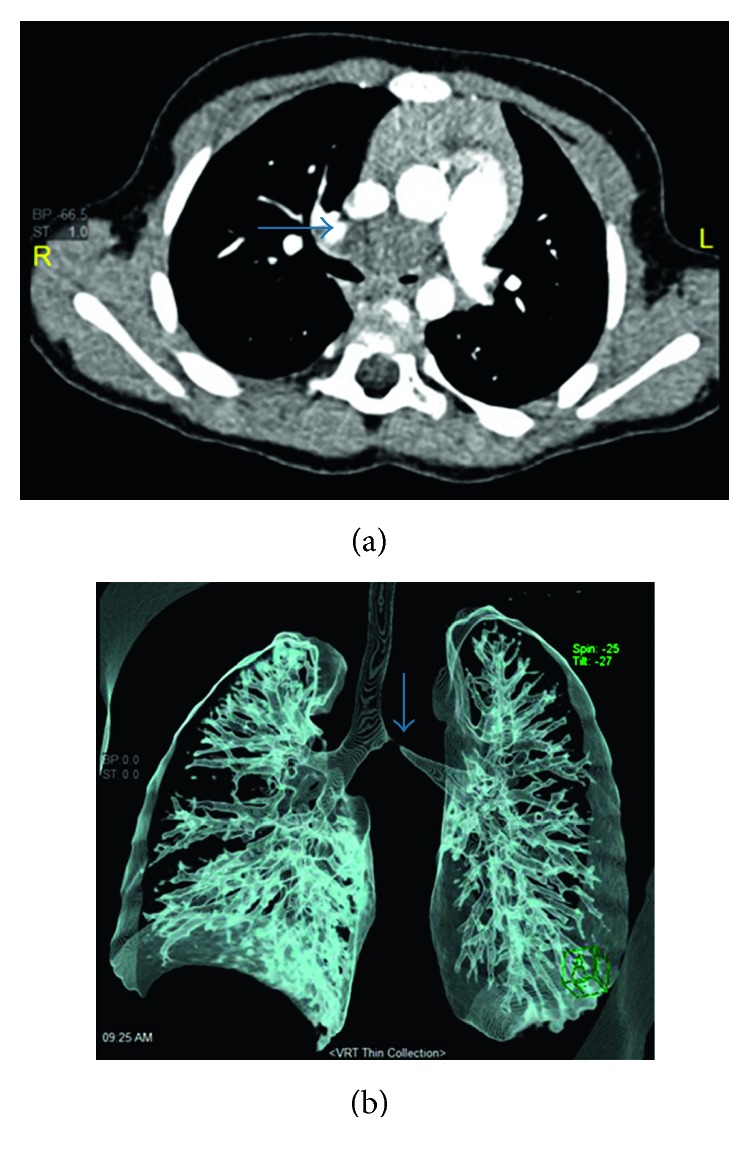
(a) CT scan of the chest showing a hypodense cystic lesion in the middle mediastinum (arrow), causing compression on the carina and the proximal aspect of the mainstem bronchi, more pronounced on the left main bronchus. (b) 3D reconstruction of the CT scan showing a significant narrowing of the left main bronchus (arrow), caused by external compression of the adjacent cystic lesion.
